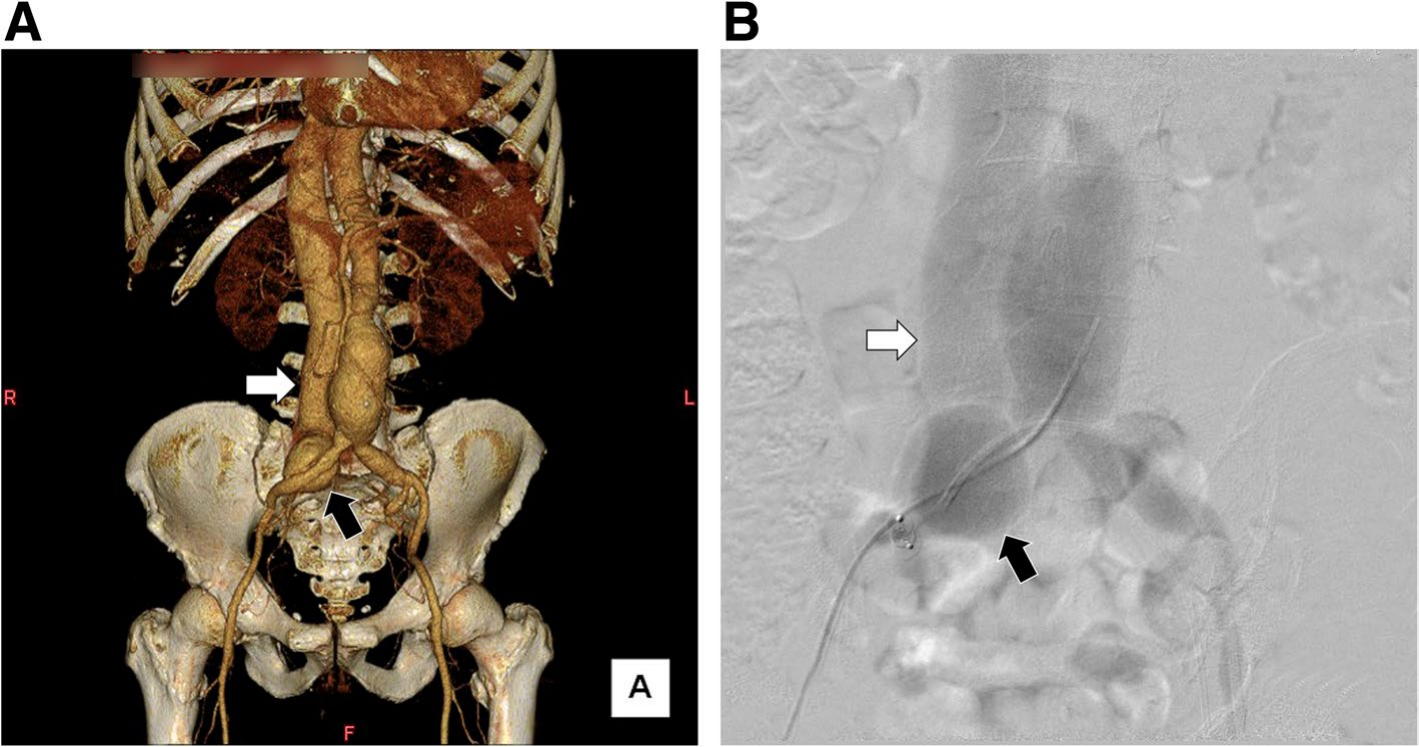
Preoperative three-dimensional reconstruction of computed tomography angiography and initial intraoperative angiography findings A Preoperative three-dimensional reconstruction of computed tomography angiography showing a 40-mm abdominal aortic aneurysm, 40-mm right common iliac arterial aneurysm with ilio-iliac fistula (black arrow), and severely dilated inferior vena cava (white arrow). B Intraoperative initial angiography showing a communication between the right iliac artery and left iliac vein (black arrow), as well as a severely dilated inferior vena cava (white arrow)
